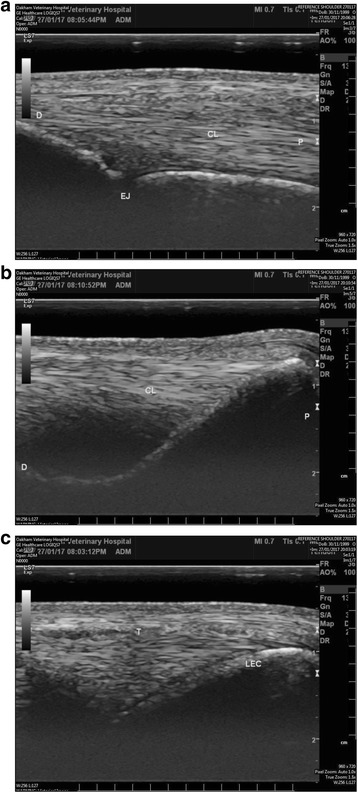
Reference ultrasonographic images. a Longitudinal ultrasonogram of the origin of the ulnaris lateralis on the lateral epicondyle of the humerus. T-ulnaris lateralis tendon; LEC-lateral epicondyle of the humerus. b Transverse ultrasonogram of the origin of the ulnaris lateralis on the lateral epicondyle of the humerus, T-Ulnaris lateralis tendon; LEC lateral epicondyle of the humerus. c Longitudinal ultrasonogram of the origin of the lateral collateral ligament of the elbow. CL lateral collateral ligament of the elbow; P- proximal; D-distal
